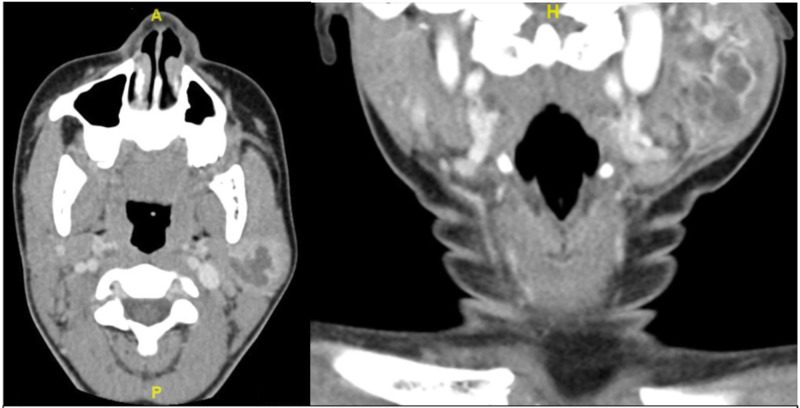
Computed tomography (CT) images of chronic sclerosing sialadenitis (CSS) of the left parotid gland in a pediatric patient Axial (left) and Coronal (right) computed tomography images of revealing loculated, cystic, left parotid mass ~6 x 4 x 3cm.
